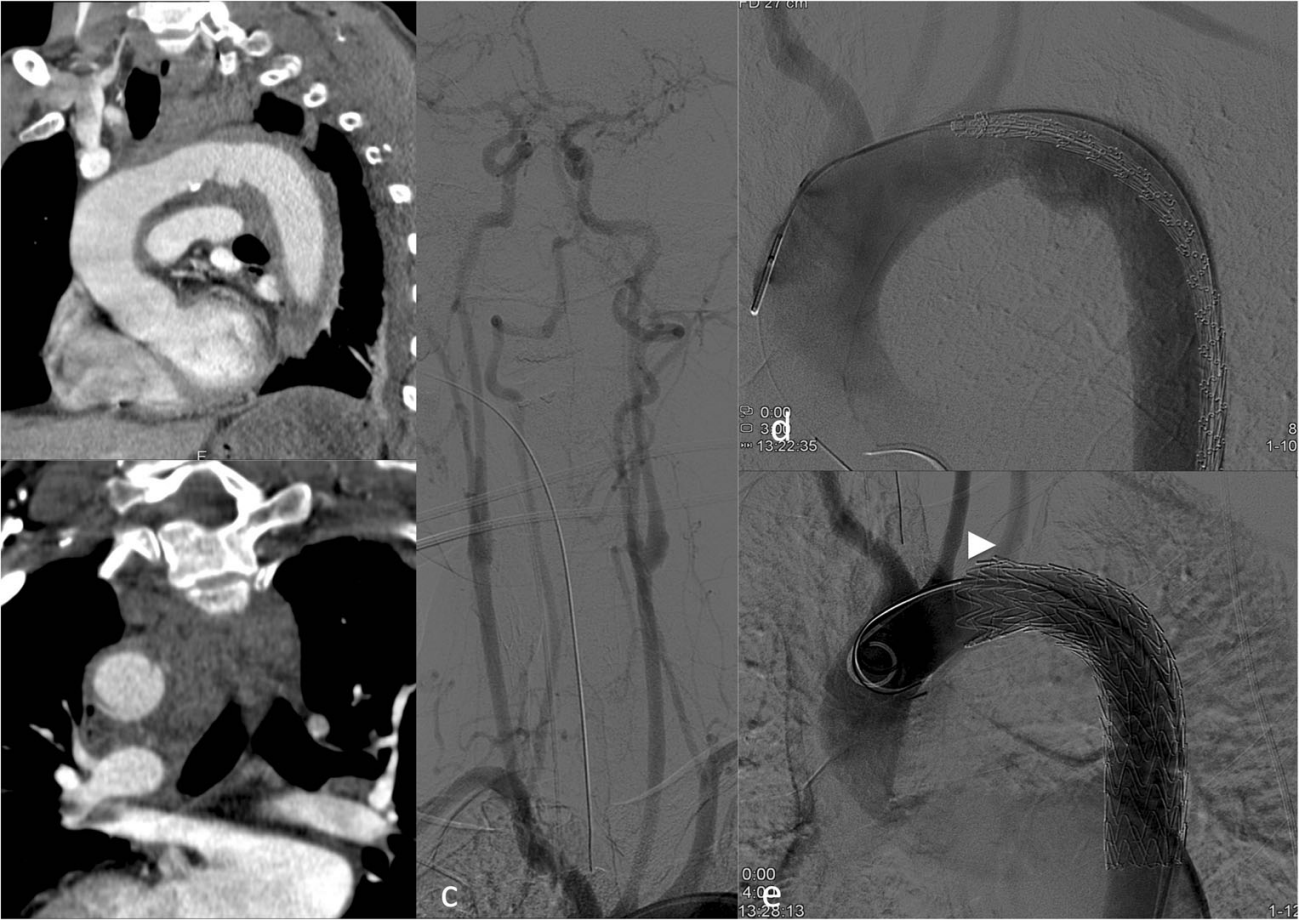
Traumatic aortic injury with stent graft implantation. A 49-year-old male patient presented with aortic trauma after a motor vehicle accident. An aortic transection with a grade 3 lesion of the proximal descending aorta (a) and accompanying mediastinal haematoma (b) is seen on a para-sagittal reformation. CTA and DSA demonstrate patency of both vertebral arteries. More pronounced pseudoaneurysm formation prior to stent graft placement and exclusion of the pseudoaneurysm after stent graft placement (e) over the left subclavian artery (*arrowhead*) that is still filling during control angiography
